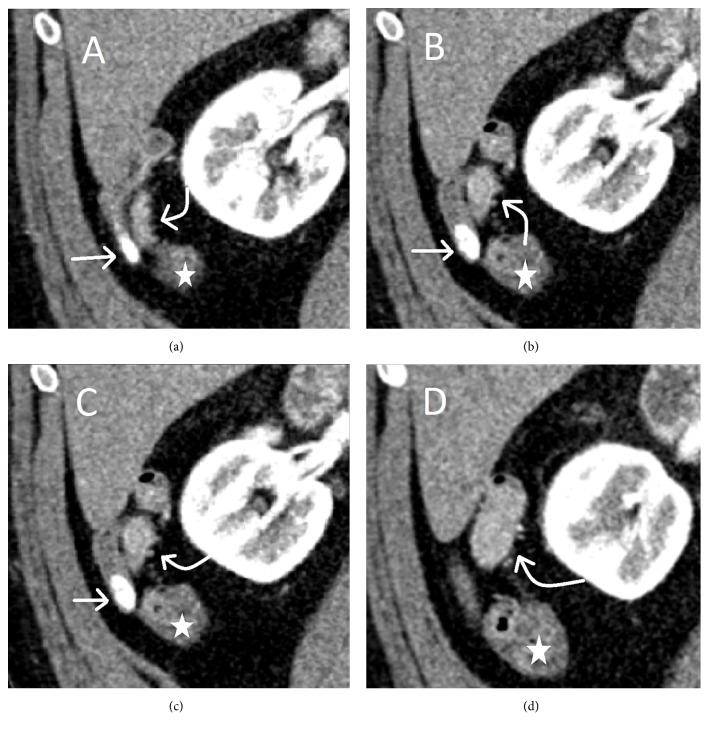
Coronal image from a contrast enhanced CT shows the appendiceal mass. At the widest portion (D) the appendix measured 16 mm in diameter. Cecum (star). Appendicolith at the tip of appendix (straight arrow). Enhancing appendiceal mass concerning mucinous tumor of the appendix (curved arrow).
